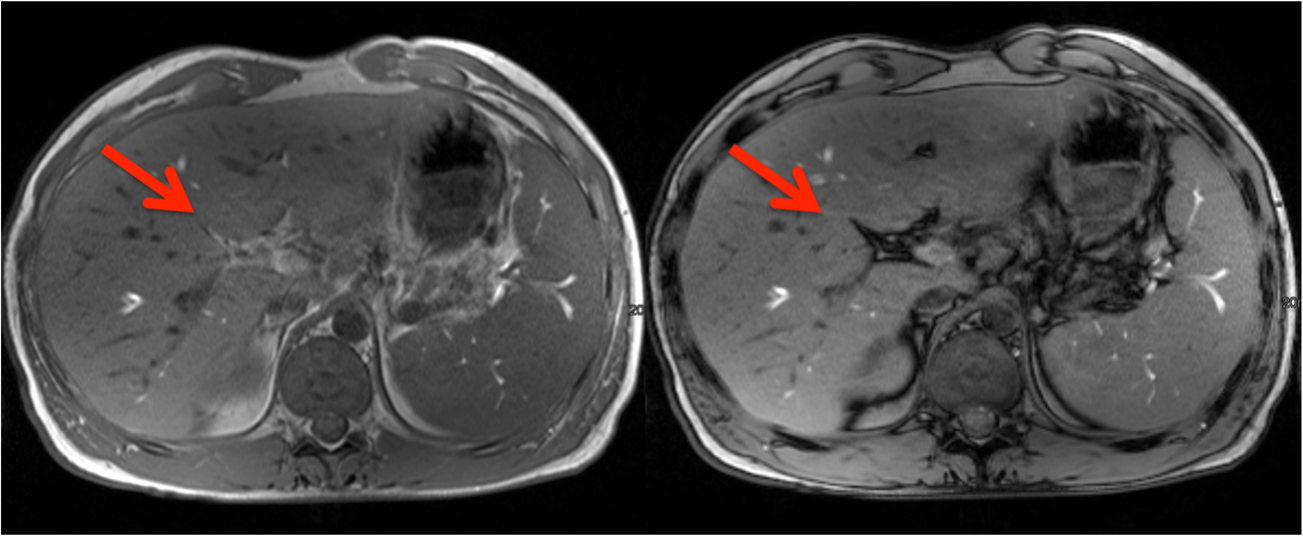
A 23-year-old male cystic fibrosis (CF) patient: T1-weighted in- and opposed-phase images demonstrate the periportal fat deposition (PPFD) as a band-like signal intensity loss on the opposed phase image compared to the in-phase image along the fissure of the porta hepatis
